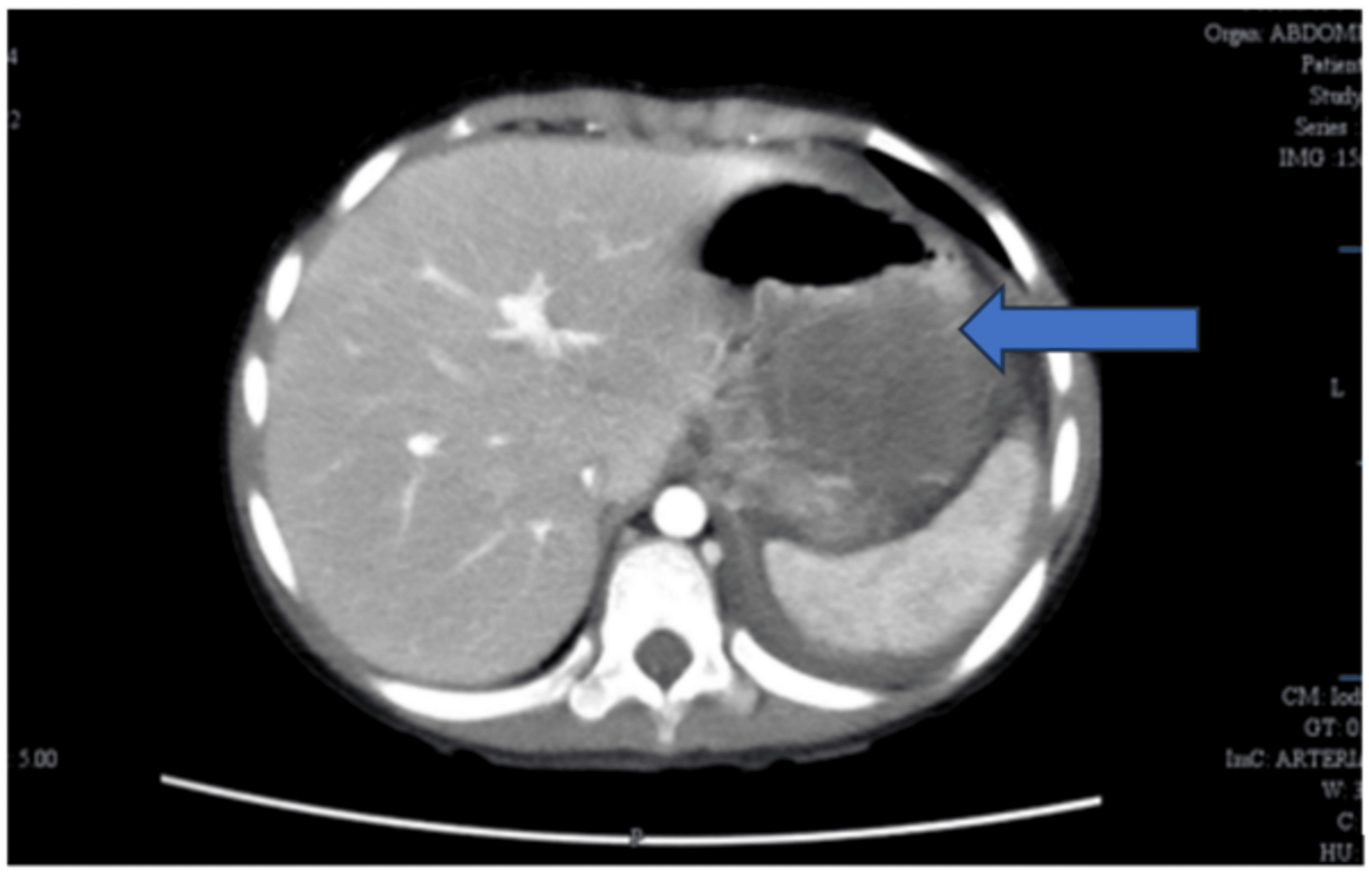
Contrast-enhanced CT scan of the abdomen (axial section) showing a well-defined mass lesion (blue arrow), adjacent to the stomach and spleen, corresponding to the clinically palpable abdominal mass CT: computed tomography
